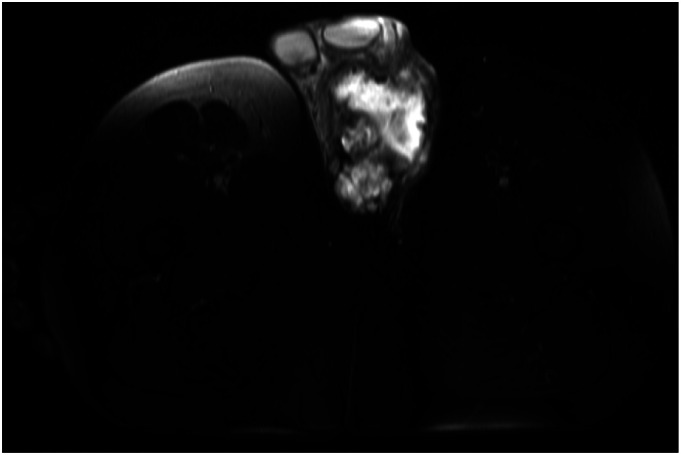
MRI showing extraskeletal myxoid chondrosarcoma in the left left groin, lateral to the left testis.
